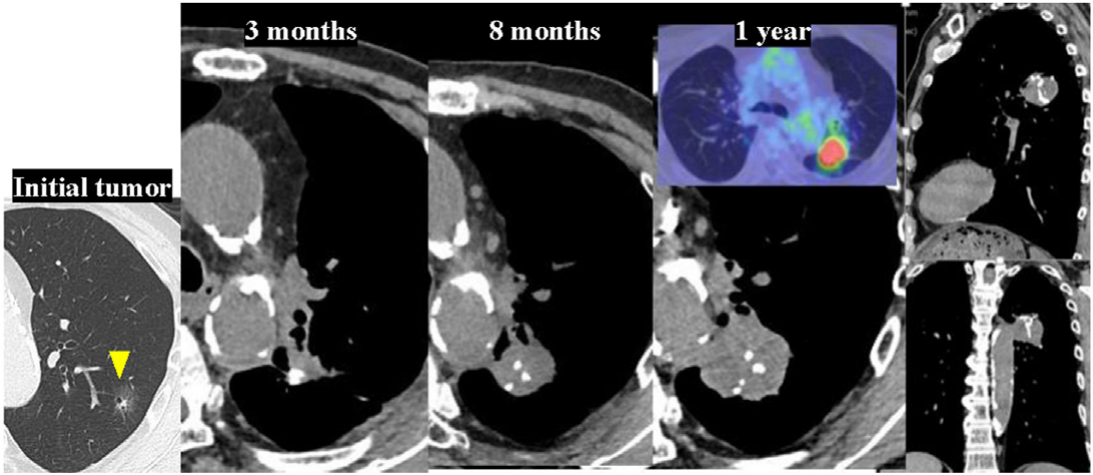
Temporal changes on chest computed tomography of the initial tumor (arrowhead) and the associated resected margins are demonstrated. The tumor at the resection margin is enlarged to a maximum diameter of 35 × 32 mm, and the maximum standardized uptake value on ^18^F-fluorodeoxyglucose positron emission tomography is 9.2.
